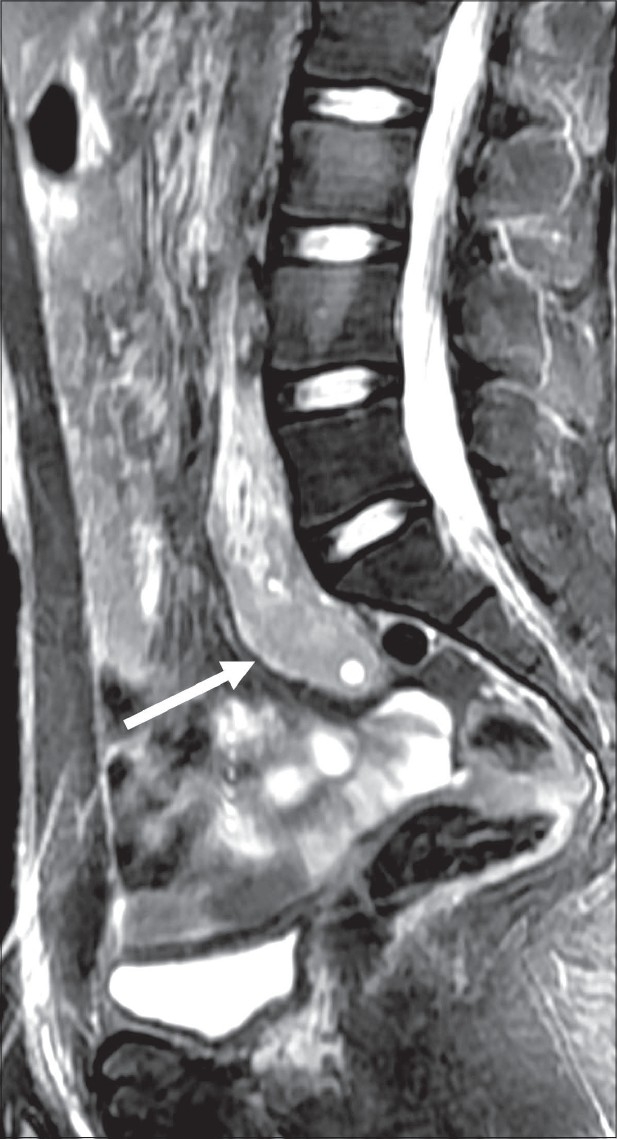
MRI of the lower abdomen and pelvis (sagittal fat-suppressed image) shows an ectopic pelvic location of the kidney (arrow). The prostate gland is not visualized
